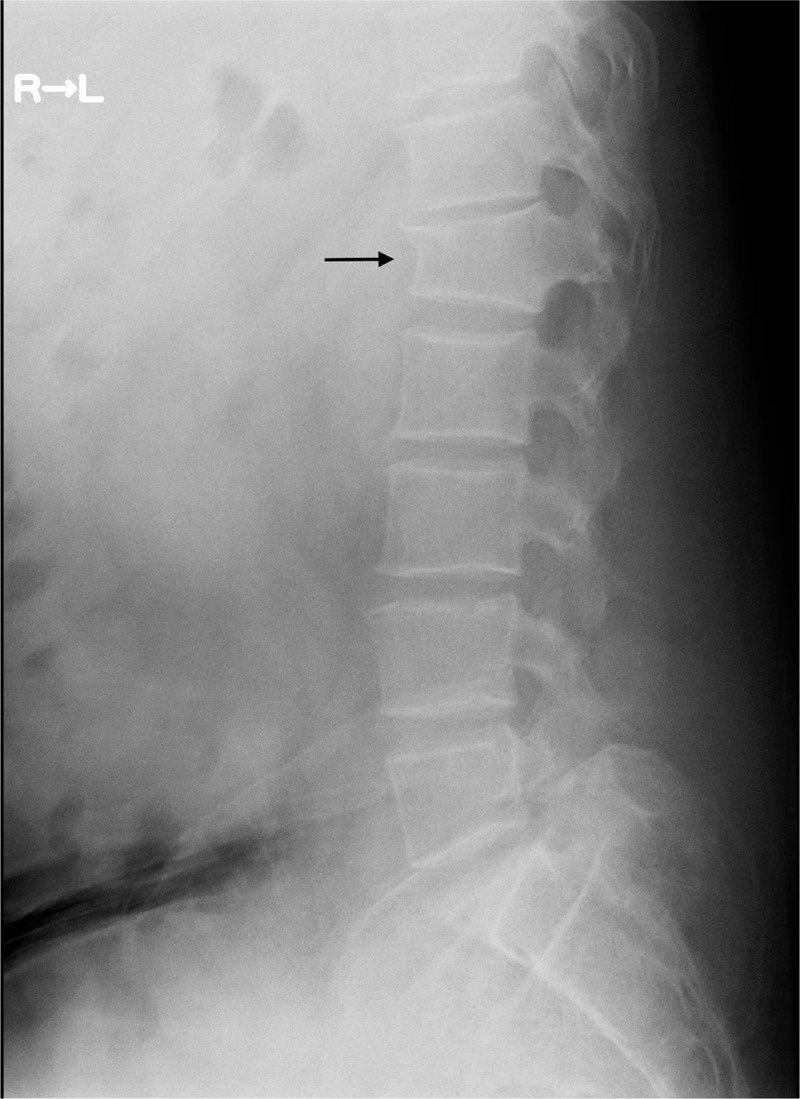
An x-ray showing L-1 compression fracture of the patient (arrow).
